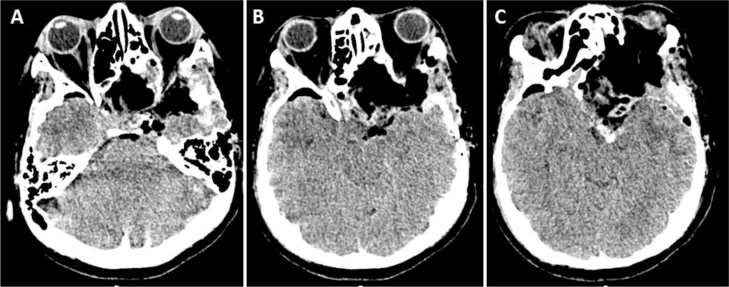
CT-scan. Axial slides (A – C) after tumor resection and post operative changes.
